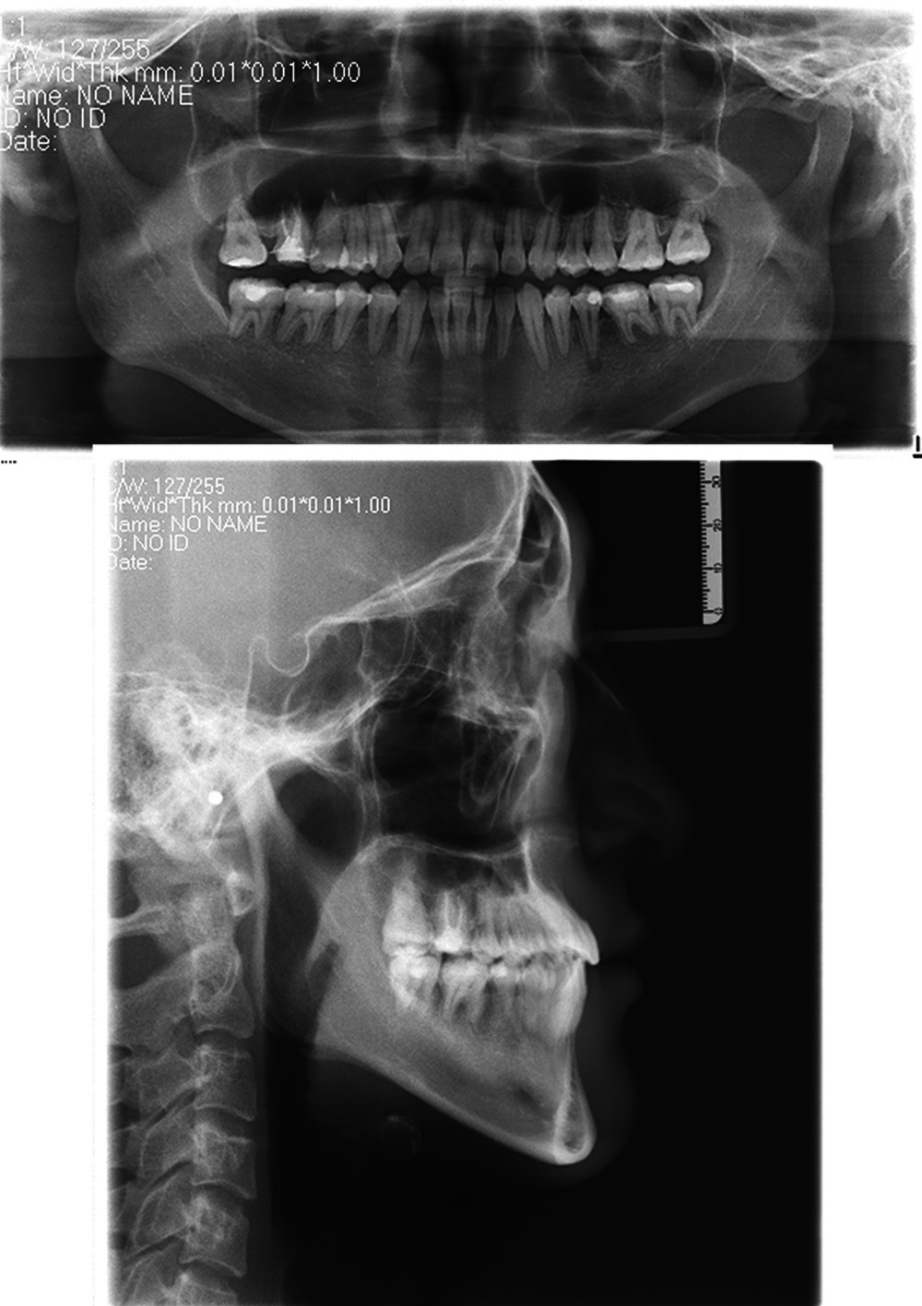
Post‐treatment panoramic radiograph and lateral cephalogram.
